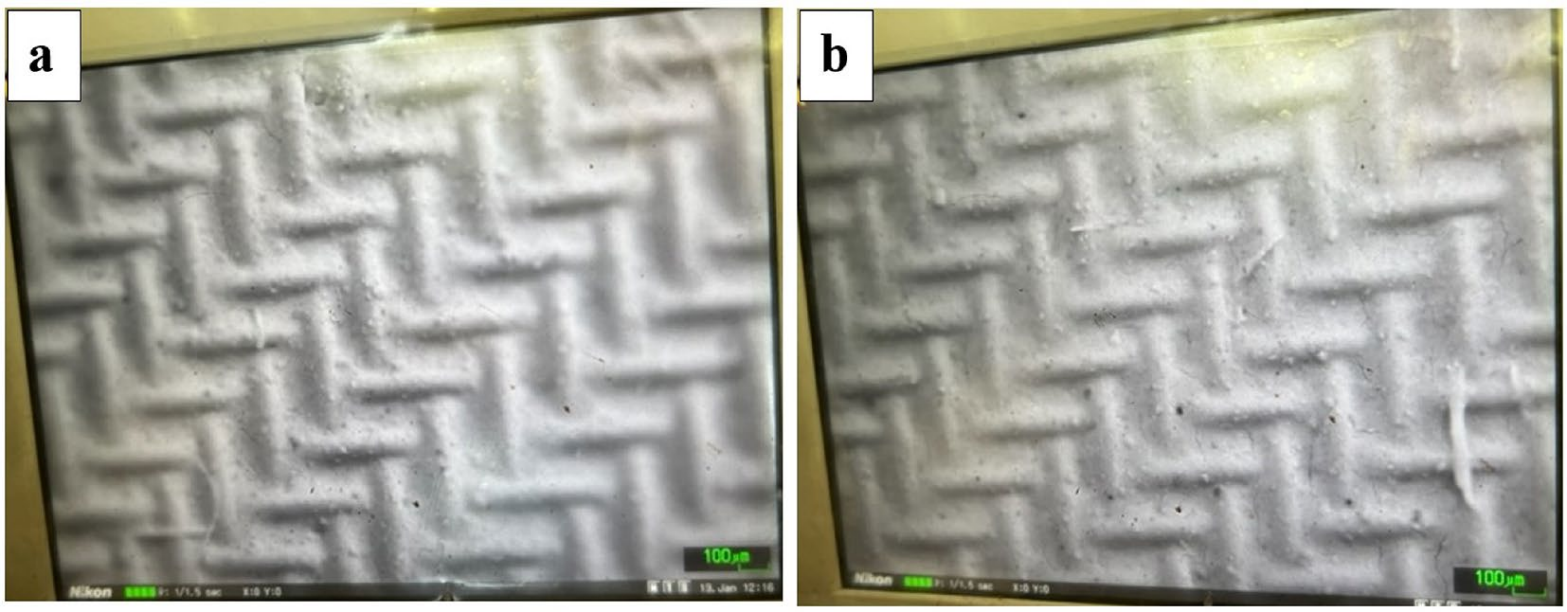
Optical images of (a) C_1_ after ultrasonication and (b) B_1_ after ultrasonication.
